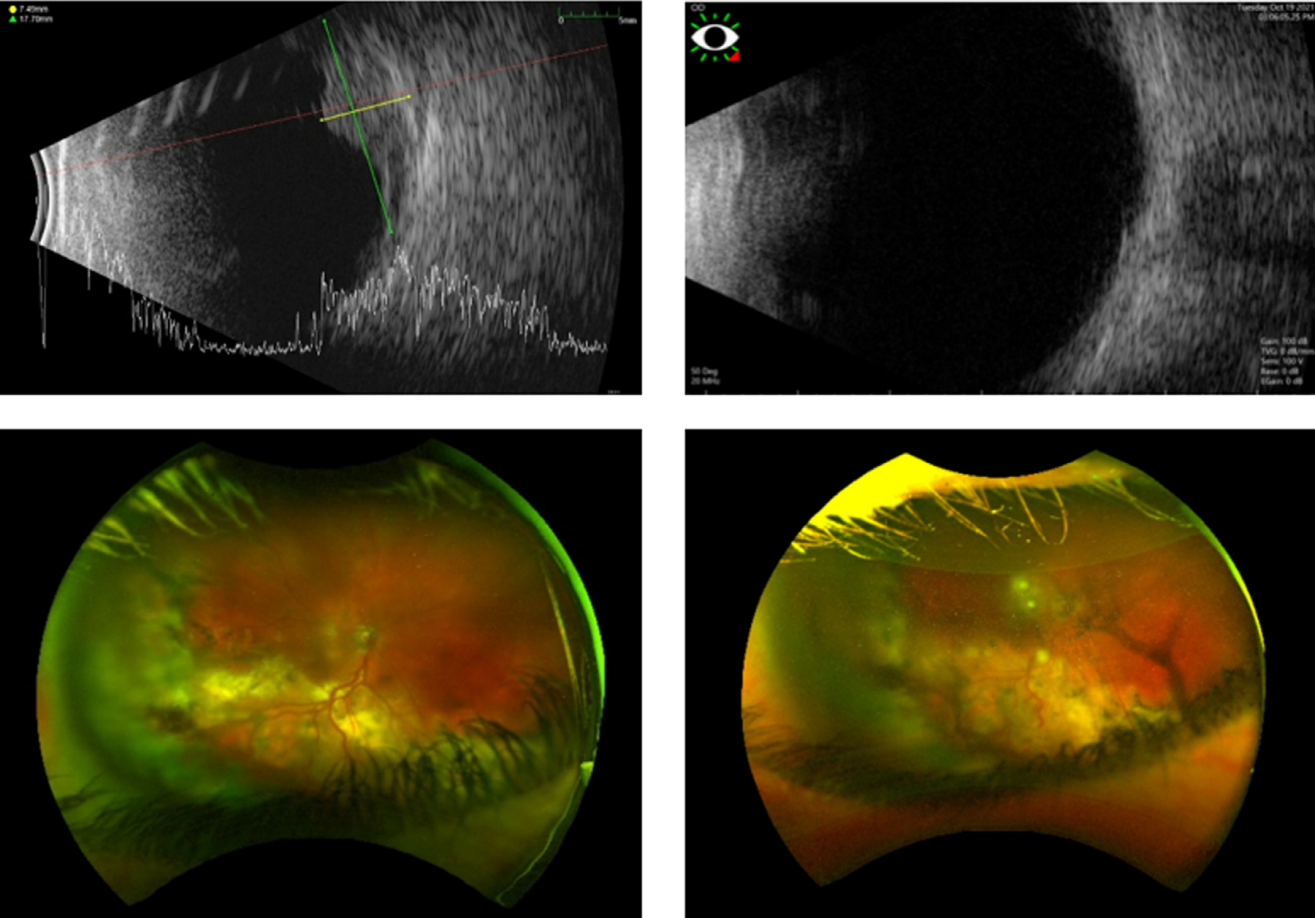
Pretreatment B-scan ultrasound and fundus image findings of right eye (left side) and 7 months after radiation therapy follow-up imaging (right side).
